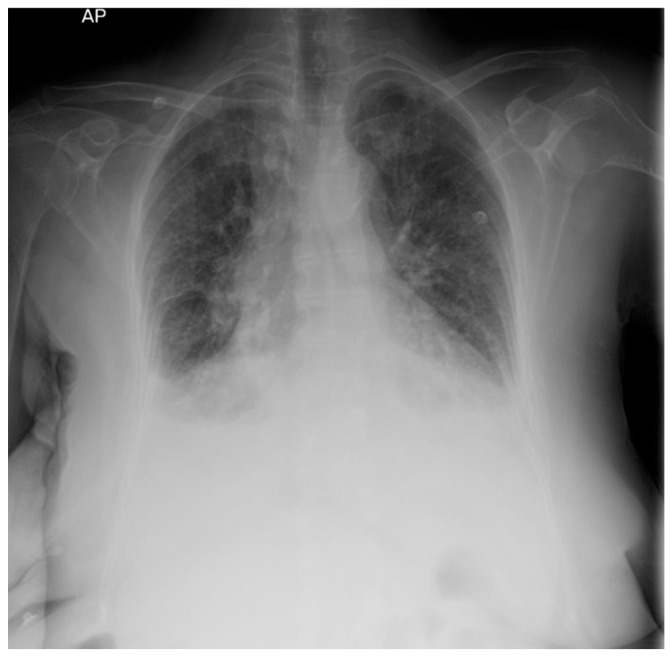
Chest X-ray: bilateral pleural effusion.
